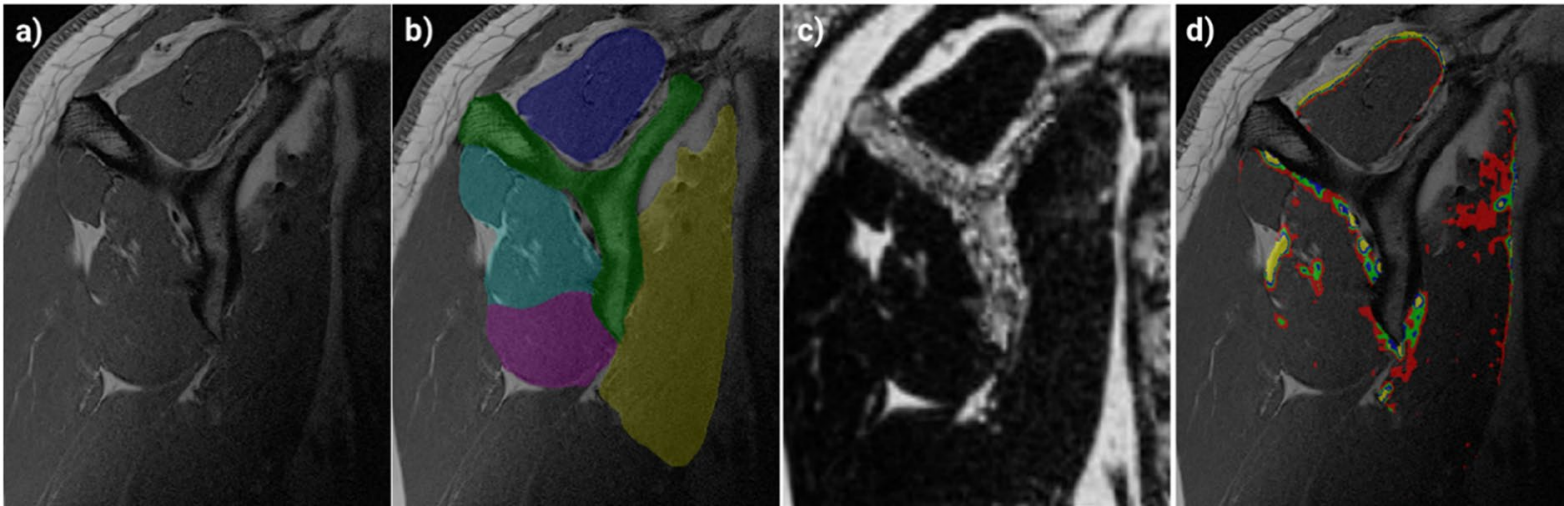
Sagittal MRI of a shoulder at Y-slice. a) T1-weighted MRI; b) automatic segmentation of the scapula (green), supraspinatus muscles (dark blue), infraspinatus muscle((cyan), teres minor muscle (magenta), subscapular muscle (yellow); c) quantitative muscle fat fraction volume, transferred from 2pDixon MRI; d) fat fraction classes inside the rotator cuff muscles, no color: <15% fat, red: 15–30% fat, green 30–45% fat, blue: 45%- 60% fat, yellow: > 60% fat.
